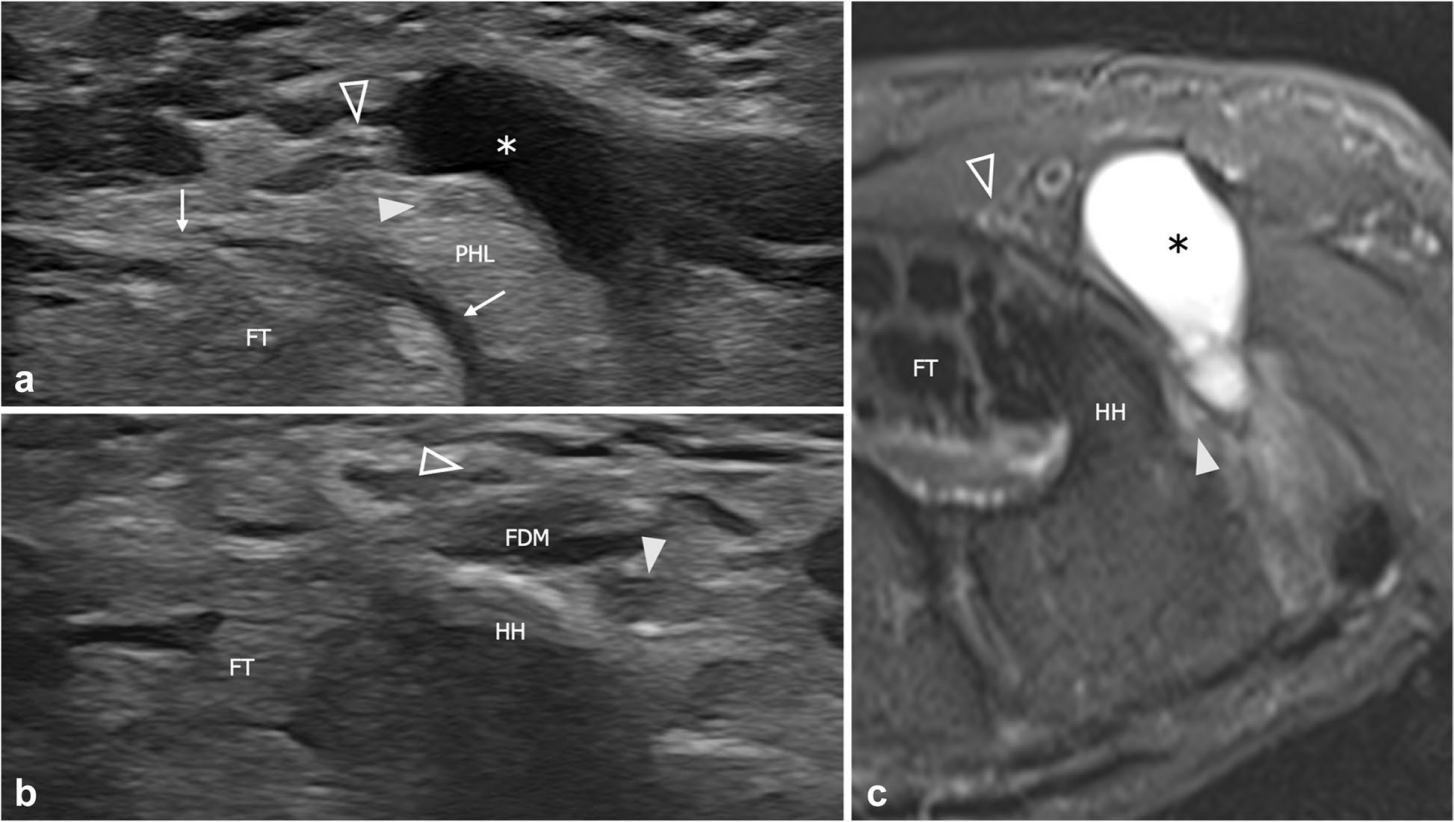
Level two extrinsic compression of the deep branch in a 61-year-old man reporting impairment in performing fine movements with the right hand. a, b Consecutive short-axis 18–5-MHz US images and c Transverse turbo Spin Echo T2-weighted MRI scan with fat saturation demonstrate a ganglion cyst (asterisk) that squeezes the deep branch (arrowhead) against the pisohamate ligament (PHL) and the hamate hook (HH). Note mild edematous changes with nerve swelling in b. The superficial branch (outlined arrowhead) is unaffected by the cyst. FT, flexor tendons; thin arrow, flexor retinaculum
